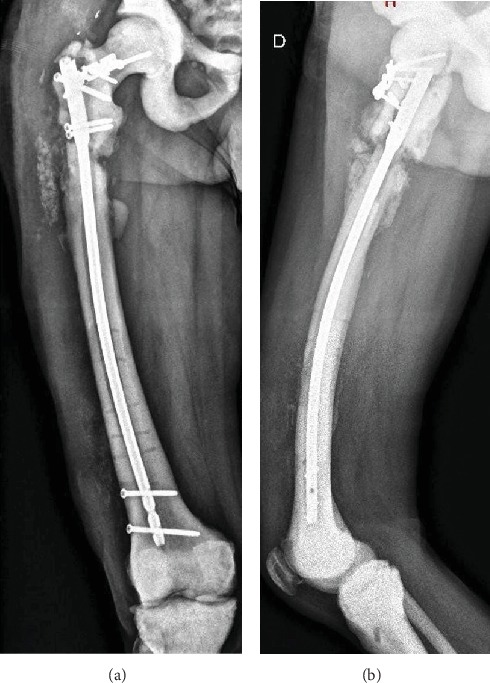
Postoperative anteroposterior (a) and lateral (b) radiographs of the third revision surgery described.
